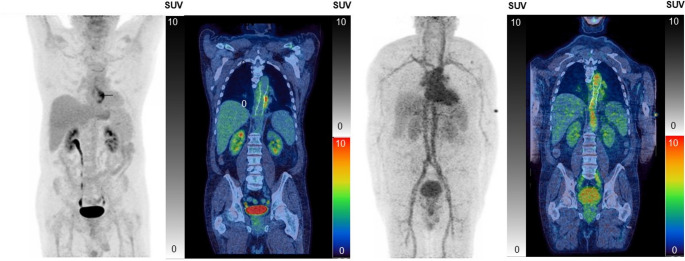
Patient 1: PET/CT scans. FDG maximum intensity projection (MIP) and coronal fused FDG images at 60 min p.i. showing focal uptake related to the aortic graft (left two panels); [^68^Ga]Ga-DFO MIP, showing high vascular uptake and coronal fused [^68^Ga]Ga-DFO without specific tracer uptake in the aortic graft at 90 min p.i. (right two panels)
